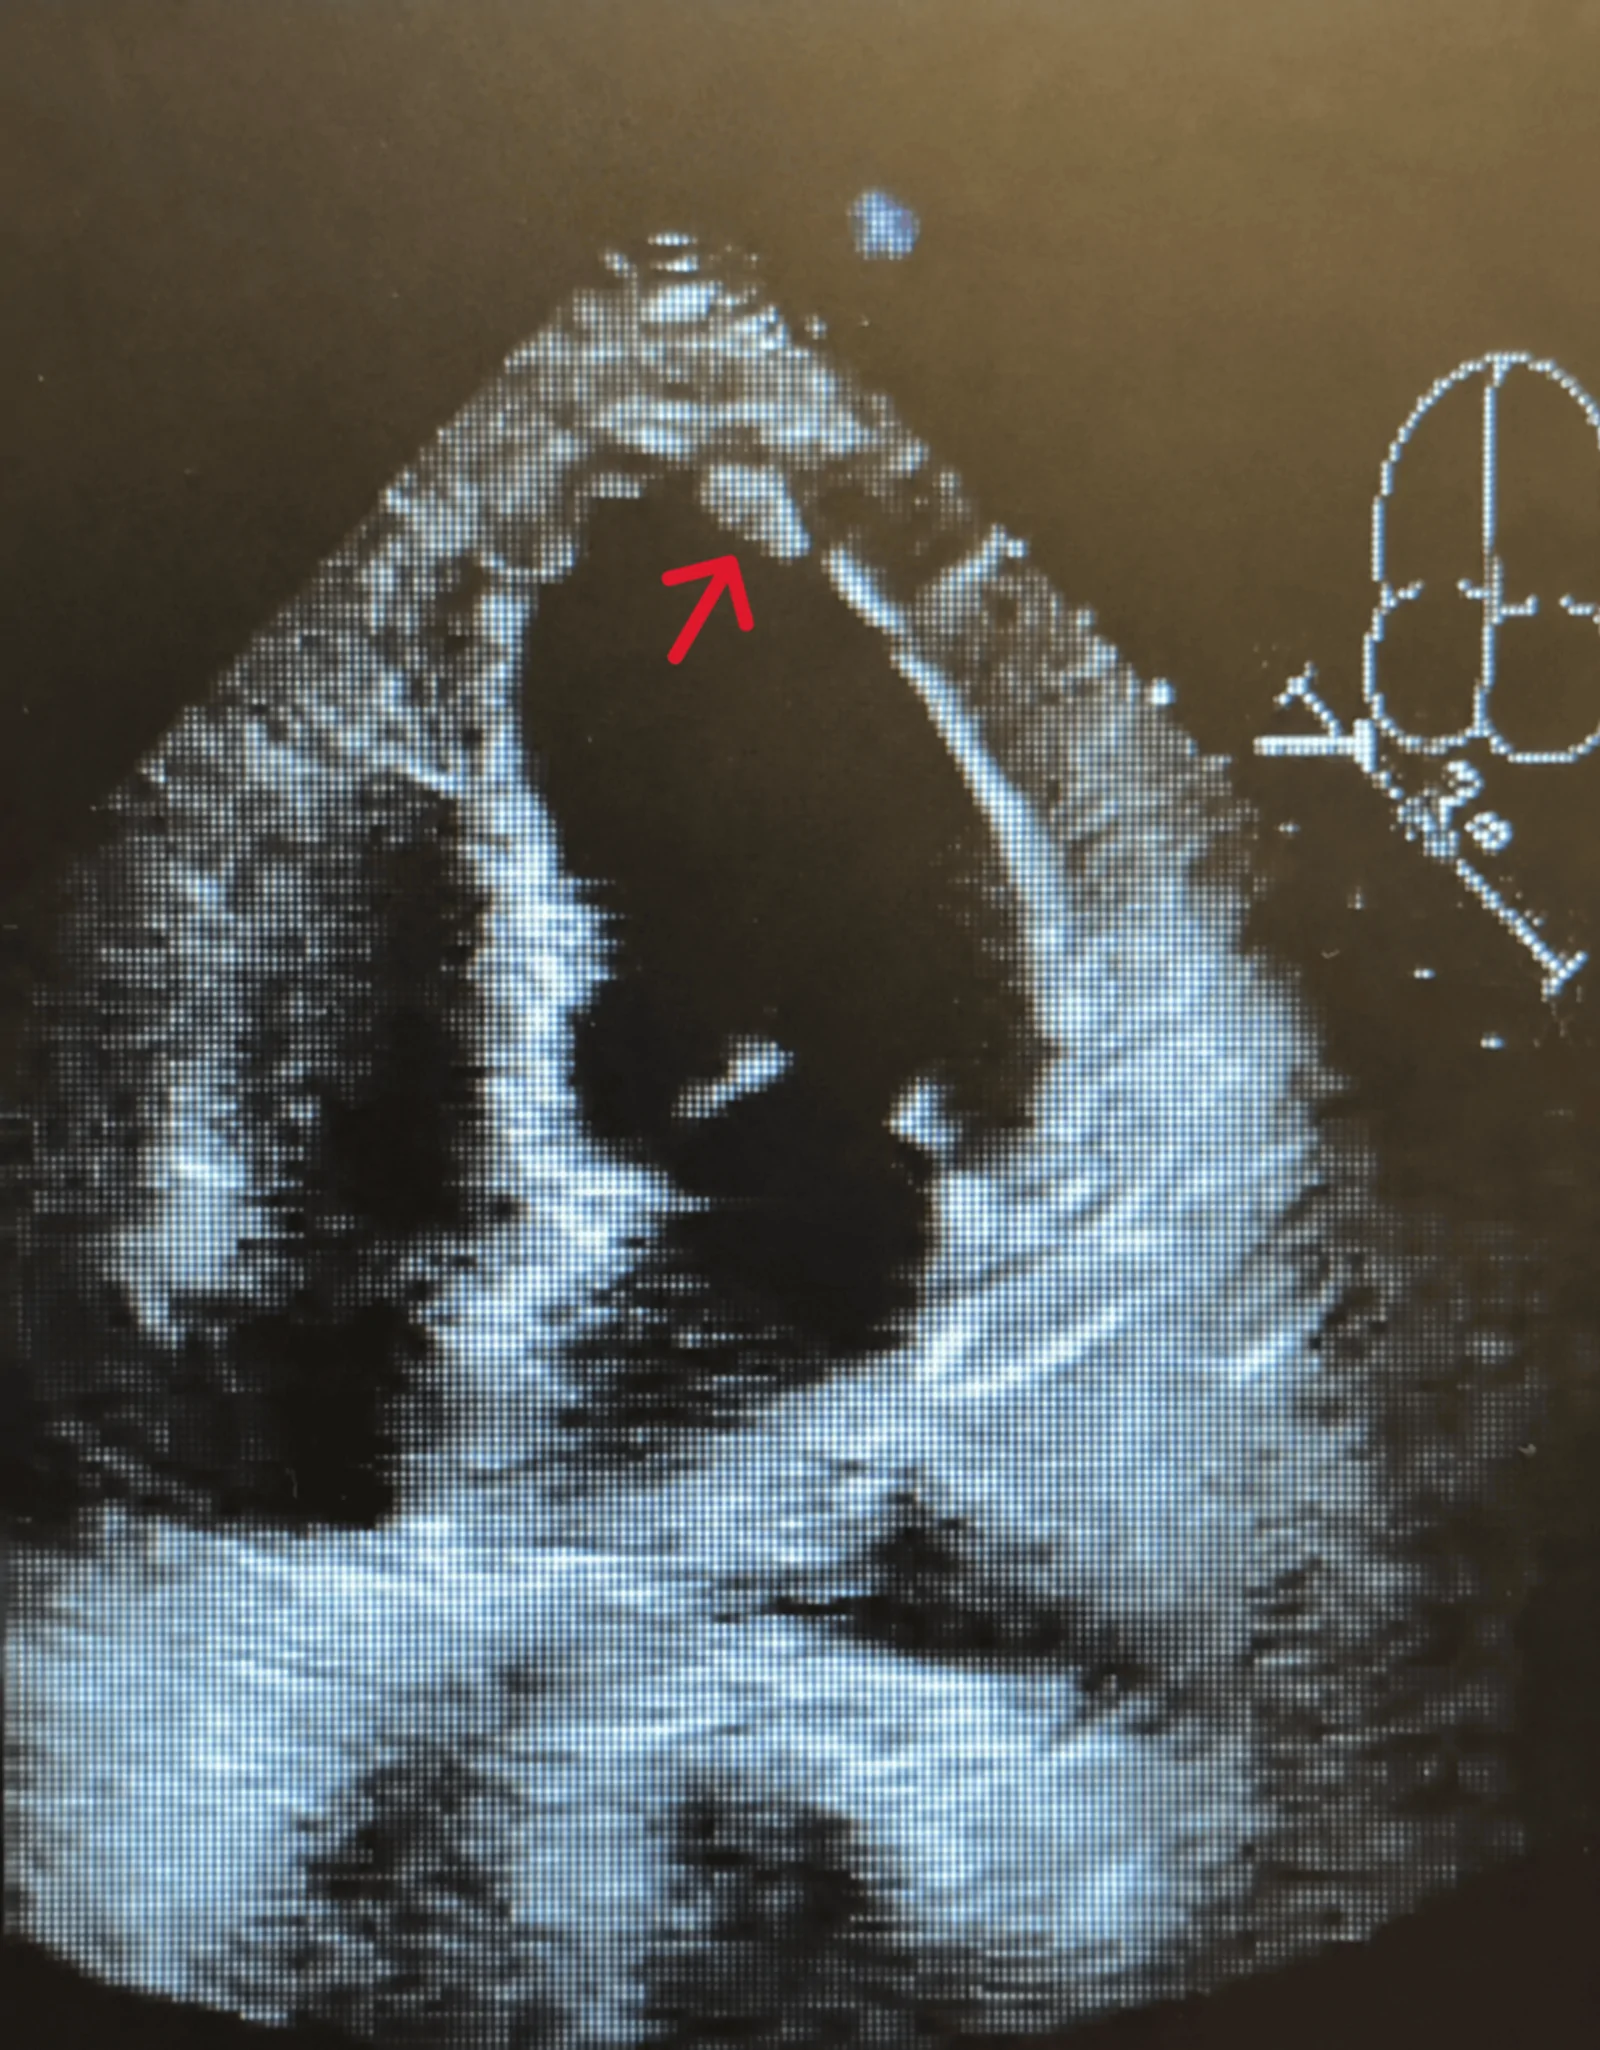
Transthoracic echocardiogram showing apical ballooning with ejection fraction (EF) of 15-20%, consistent with Takotsubo cardiomyopathy and presence of LV mural thrombus (red arrow)
